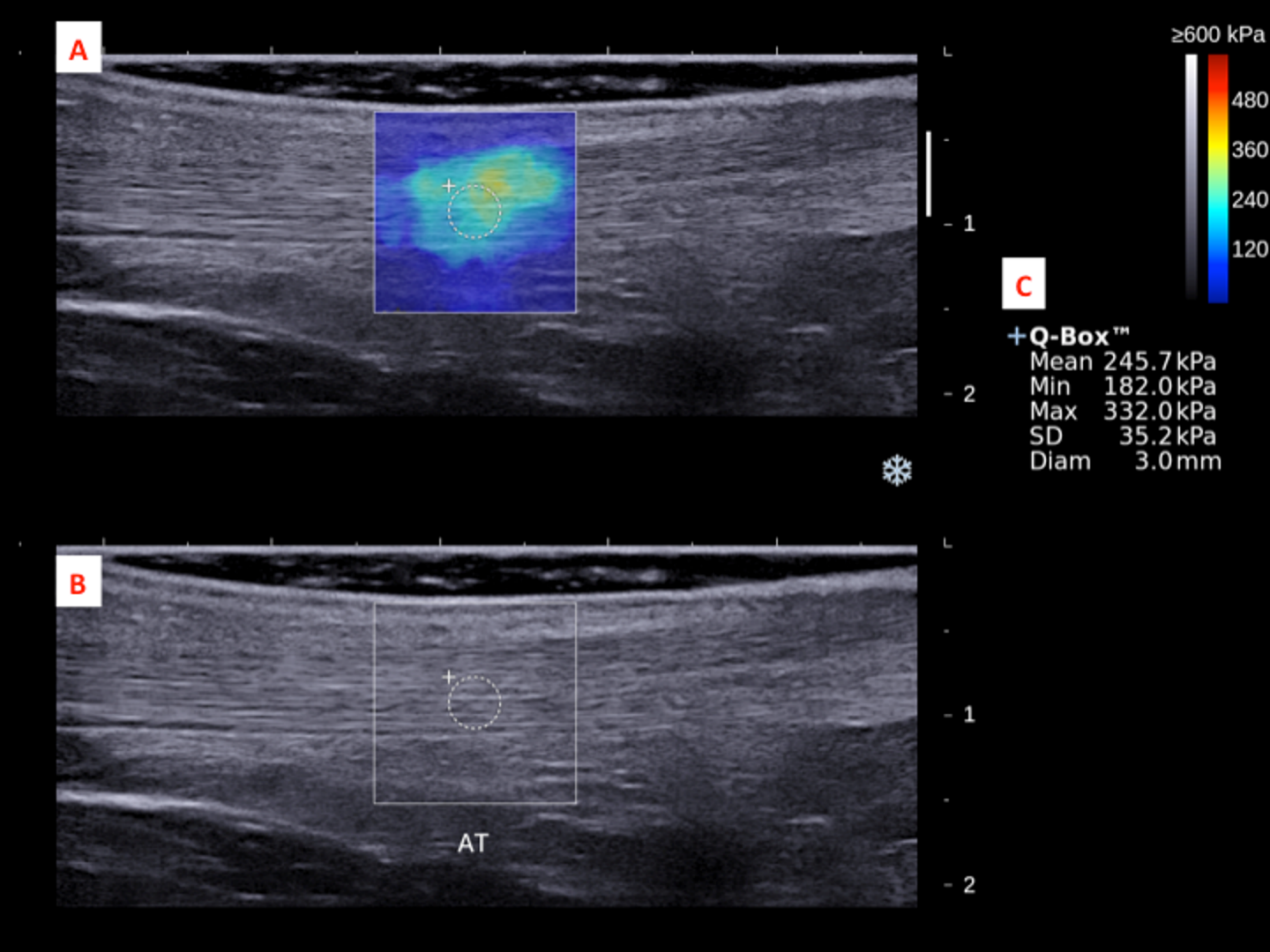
A shear wave elastogram (A) and the corresponding grey-scale ultra-sonogram (B) in the longitudinal axis of an Achilles tendon (AT) on the dominant leg of a subject after a heel drop exercise. (A) Q-box with a diameter of 3-mm was placed in the corresponding B-mode image (B) along the longitudinal axis of the AT for the tendon stiffness assessment. Different stiffness parameters were shown in (C).
